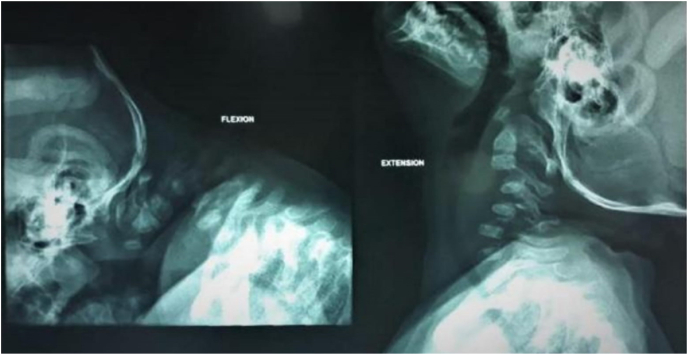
Dynamic Neck X-ray (Flexion and extension) showing cervical kyphosis, anterior wedging of C3, C4 associated with instability.
